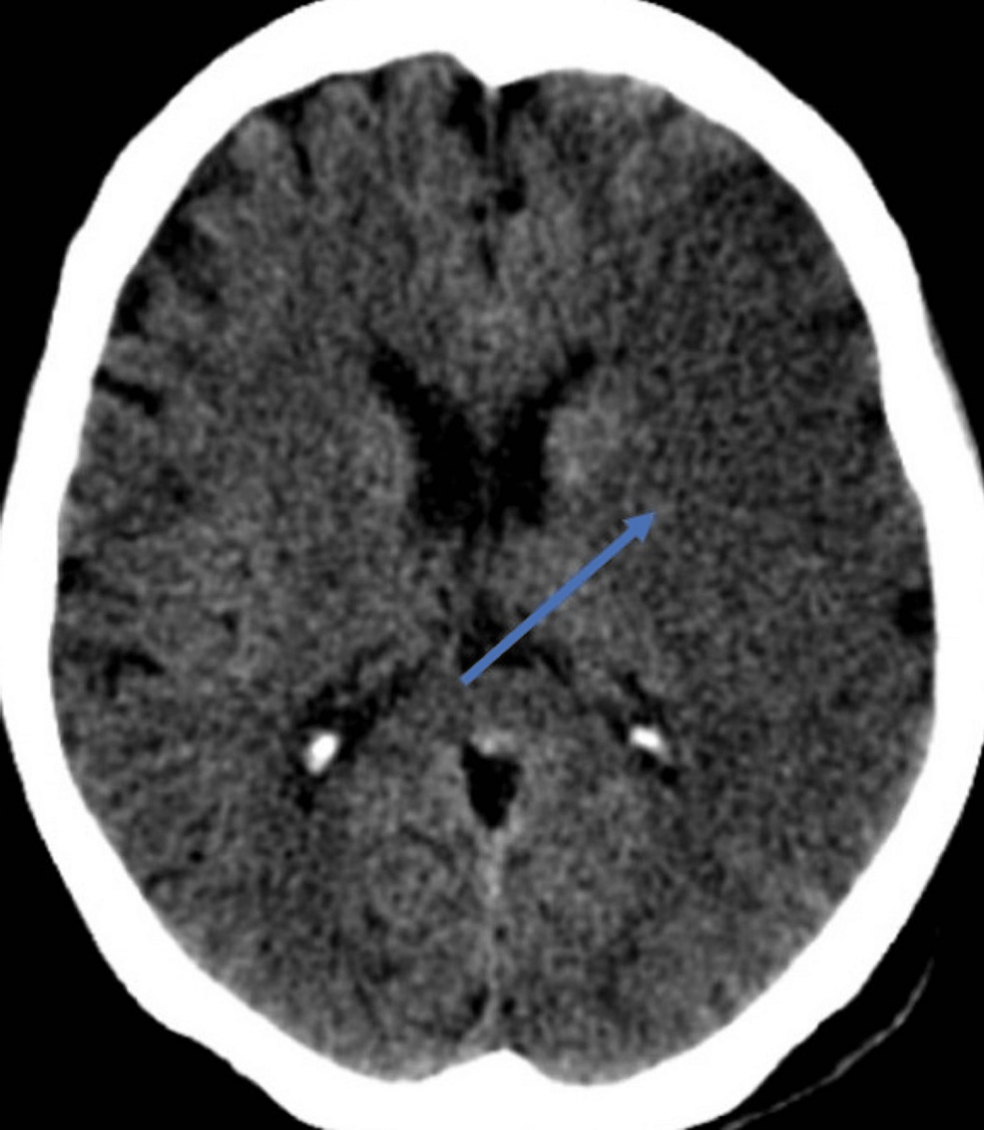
Brain CT showing hyperdense areas in the left cerebral hemisphere CT: computed tomography
